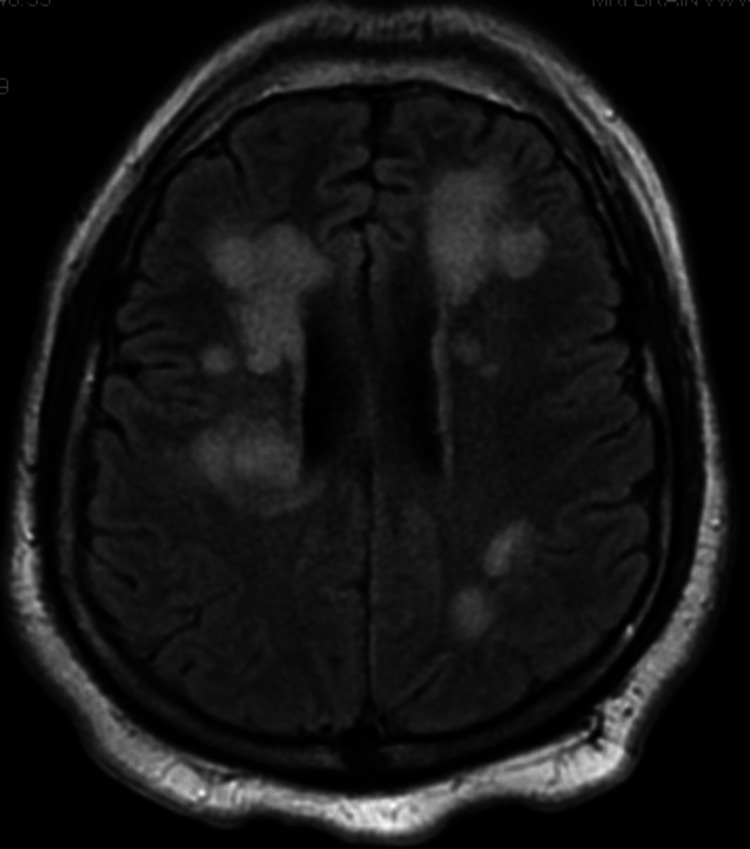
Initial MRI of the brain taken early in the patient’s hospital stay Multiple areas of hyperintensity were noted but without significant mass effect.
